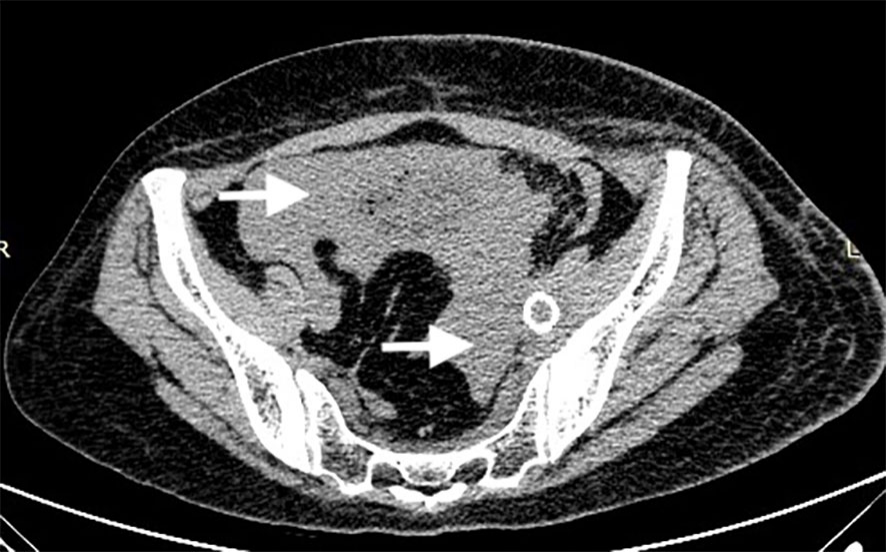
Three days after the procedure, a CT scan revealed retroperitoneal hematoma and blood and fluid accumulation in the abdominopelvic cavity. (white arrow).
